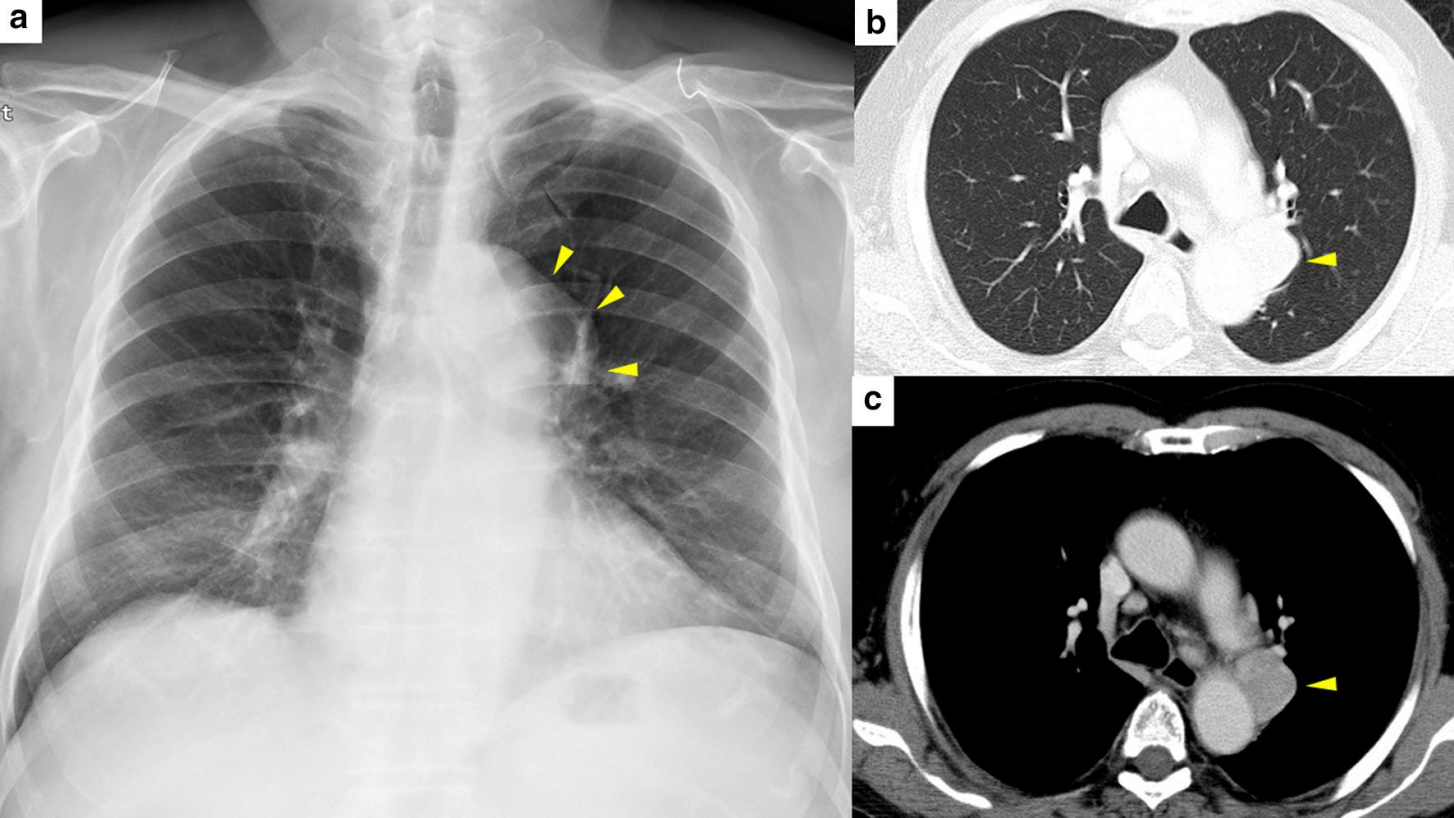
Preoperative imaging. a Chest X-ray showed an abnormal mass in the left mediastinum (yellow arrowheads). b Enhanced computed tomography of lung field (yellow arrowheads). c Enhanced computed tomography of mediastinal field; the left mediastinal mass was a homogenous round-shaped 3.8 × 2.4 cm in diameter (yellow arrowheads)
